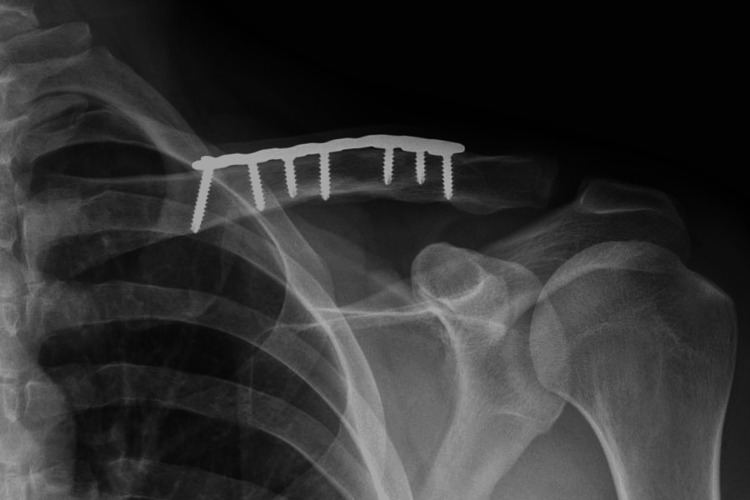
Postoperative radiograph showing a healed fracture in anatomic alignment after osteosynthesis using a superior plate fixated by seven screws.
